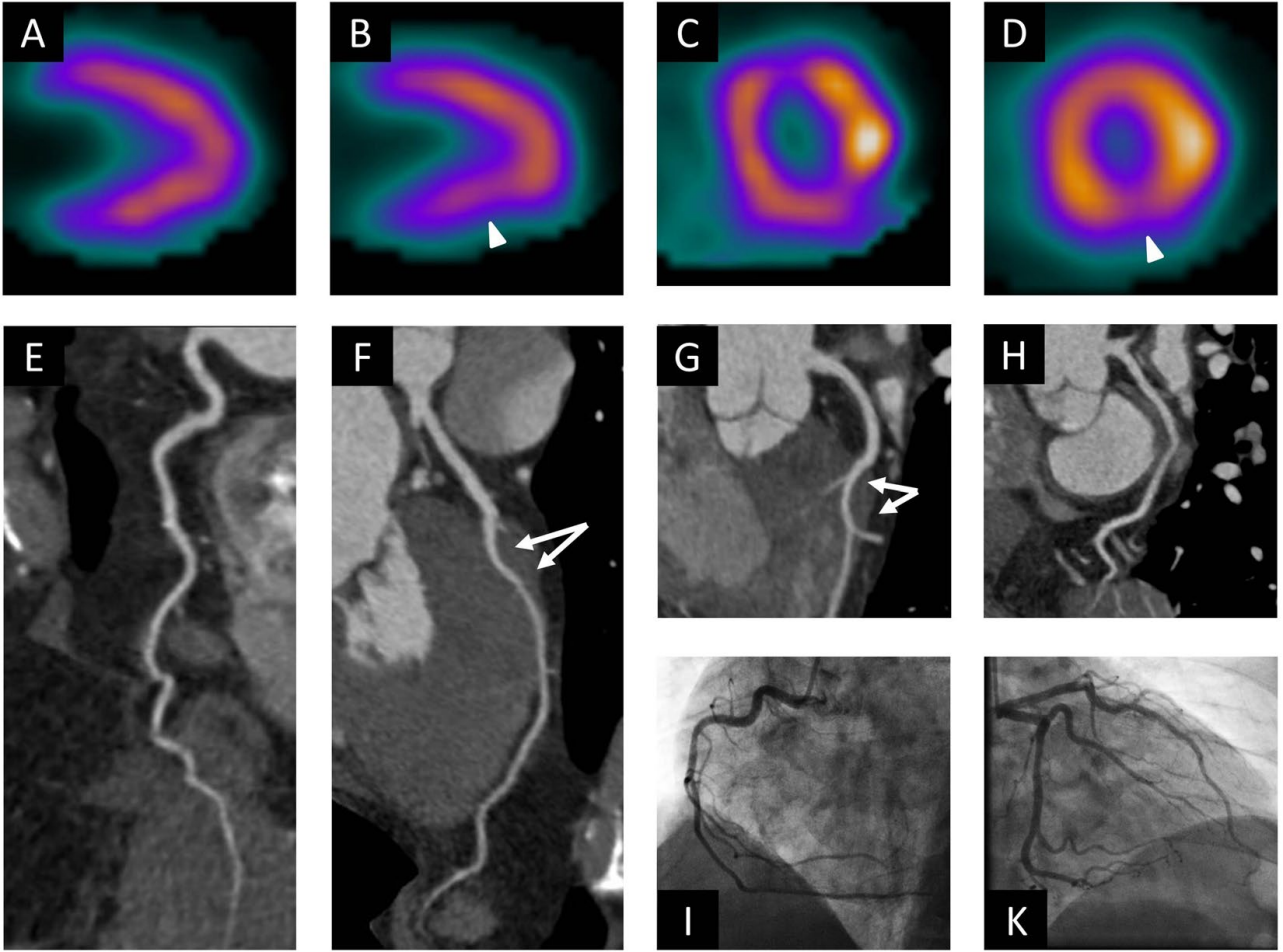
Example of a true negative CCTA examination. This 58 year old male patient underwent stress nuclear myocardial perfusion imaging because of chest pain and dyspnea on exertion. Stress images in the vertical long axis (B) and horizontal short axis views (D) are suggestive of decreased perfusion to the inferior wall of the left ventricle (arrow heads), which are at significantly reversible at rest without extracardiac activity (A,C). The examination was thus interpreted as concerning for inferior wall ischemia. At CCTA, curved multiplanar reformats of the right coronary artery (E), left anterior descending artery (F,G) and circumflex artery (H) demonstrate no evidence of stenosis. Myocardial bridging of the left anterior descending artery with a relatively long intra-myocardial course as a potential cause of the patient’s symptoms is noted (arrows in F,G). On catheter angiograms (I,K), there is no evidence of coronary artery disease. CCTA = coronary computed tomography angiography.
